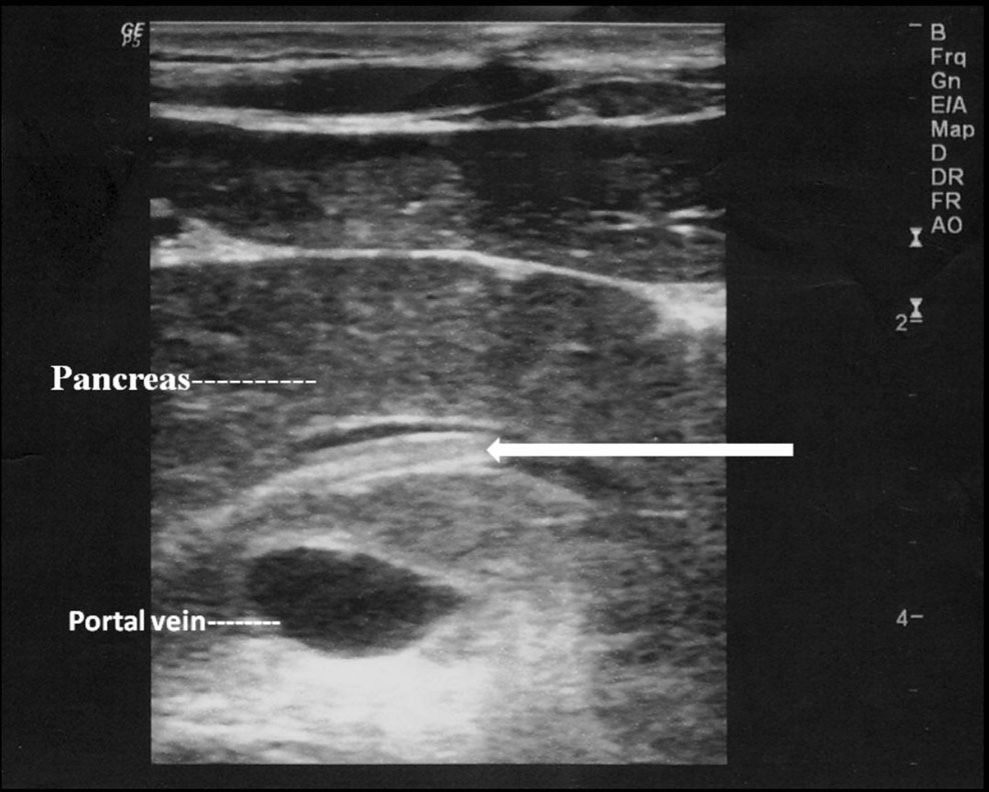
A 15-year-old male presented with acute epigastric pain and elevated pancreatic enzymes. High resolution ultrasound using linear array transducer shows ascaris with triple line within in the dilated main pancreatic duct (*long white arrow*) with pancreatitis
